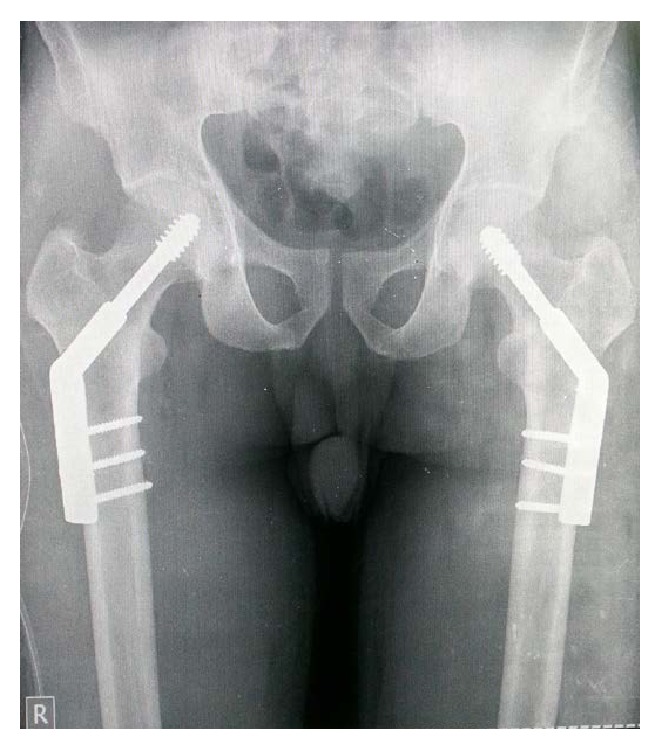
Immediate postoperative X-ray of bilateral hip joint with pelvis showing 3 holed dynamic hip screw implant in situ.
